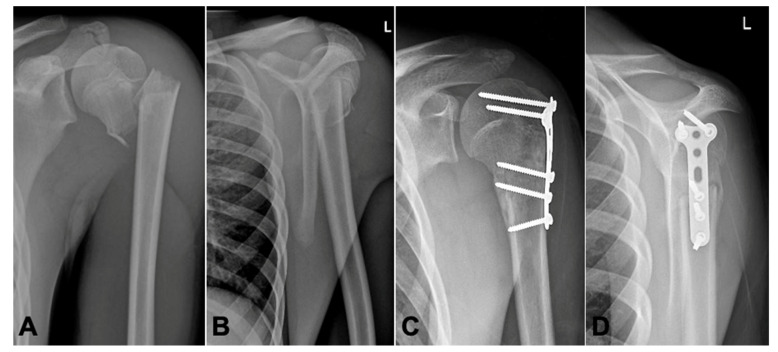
Radiographic shoulder views (Pat. N° 4) (ap/Neer). (A,B): Dorsally displaced humeral head. (C,D): 10 weeks after open reduction and plate fixation.
